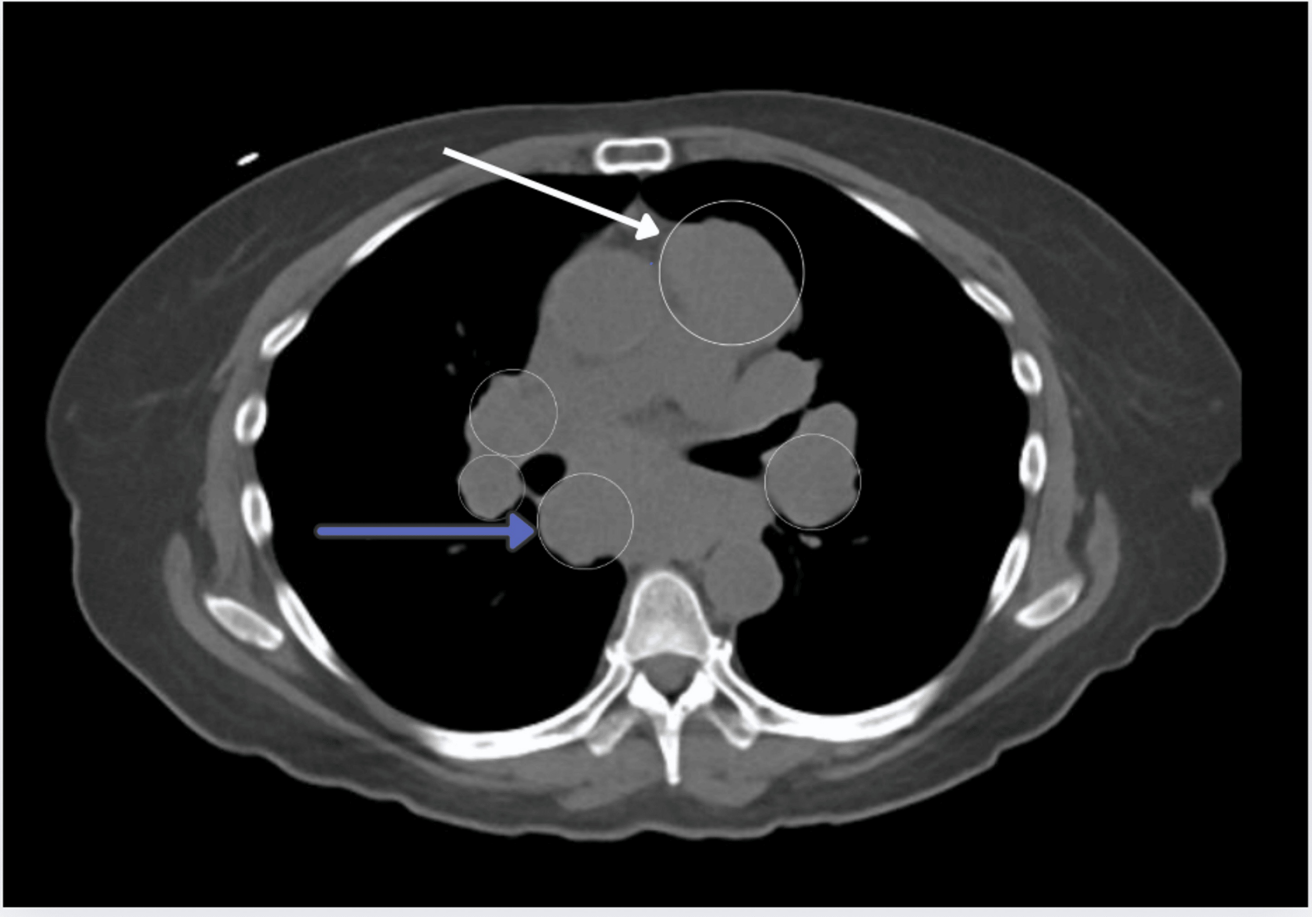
CT scan showing mediastinal (white arrow) and hilar (purple arrow) lymphadenopathy, which are suggestive of a lymphoproliferative disorder. The presence of mesenteric lymphadenopathy also raises concern for an underlying malignancy CT: computed tomography
